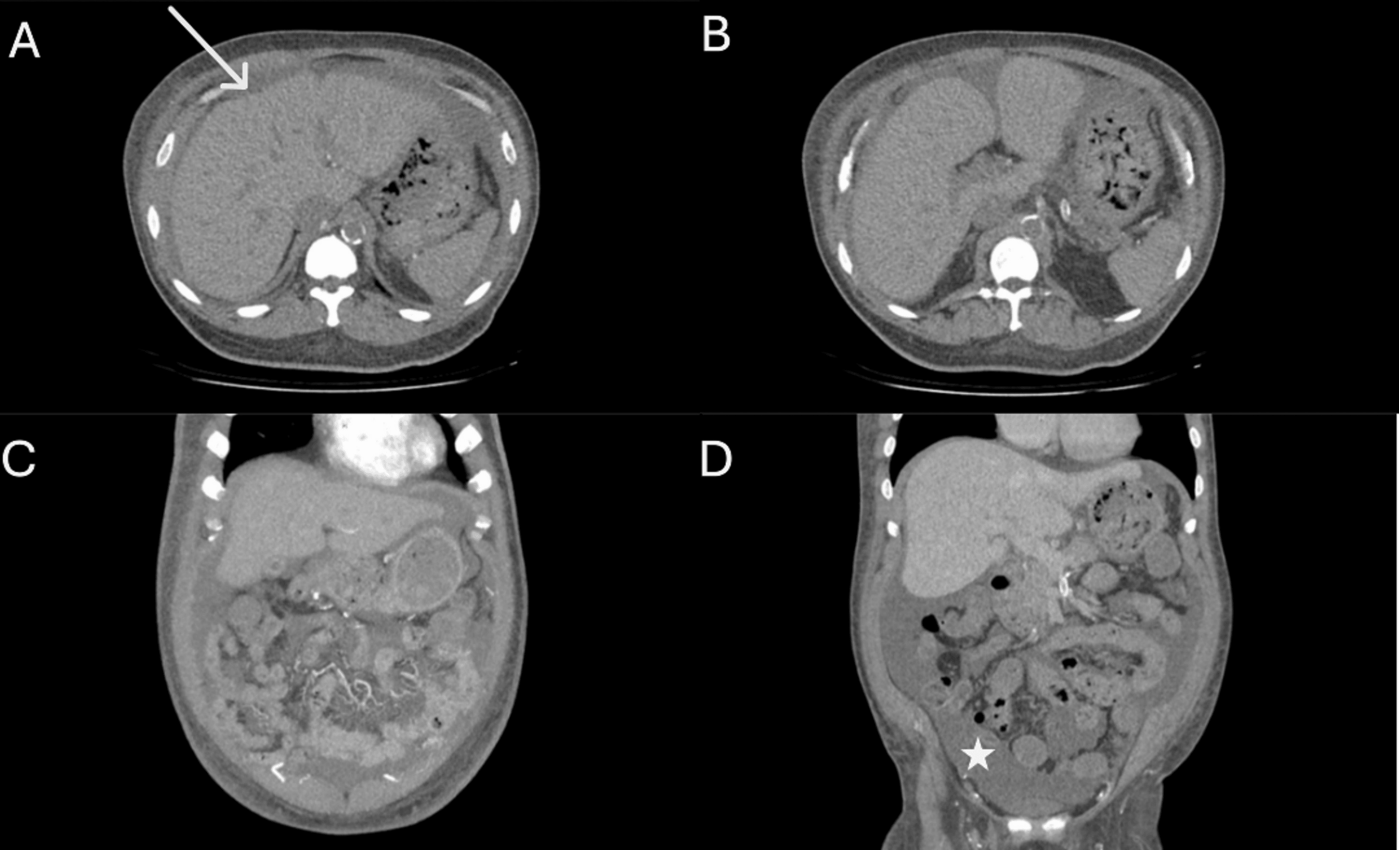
Computed tomography of the abdomen Transverse (A and B) and coronal (C and D) abdominal computed tomography axes demonstrating mild hepatomegaly and mild nodular liver contour (arrow) and ascites (asterisk). The liver findings, along with the patient’s clinical presentation, subsequently led to a liver biopsy, which did not reveal cirrhosis.
